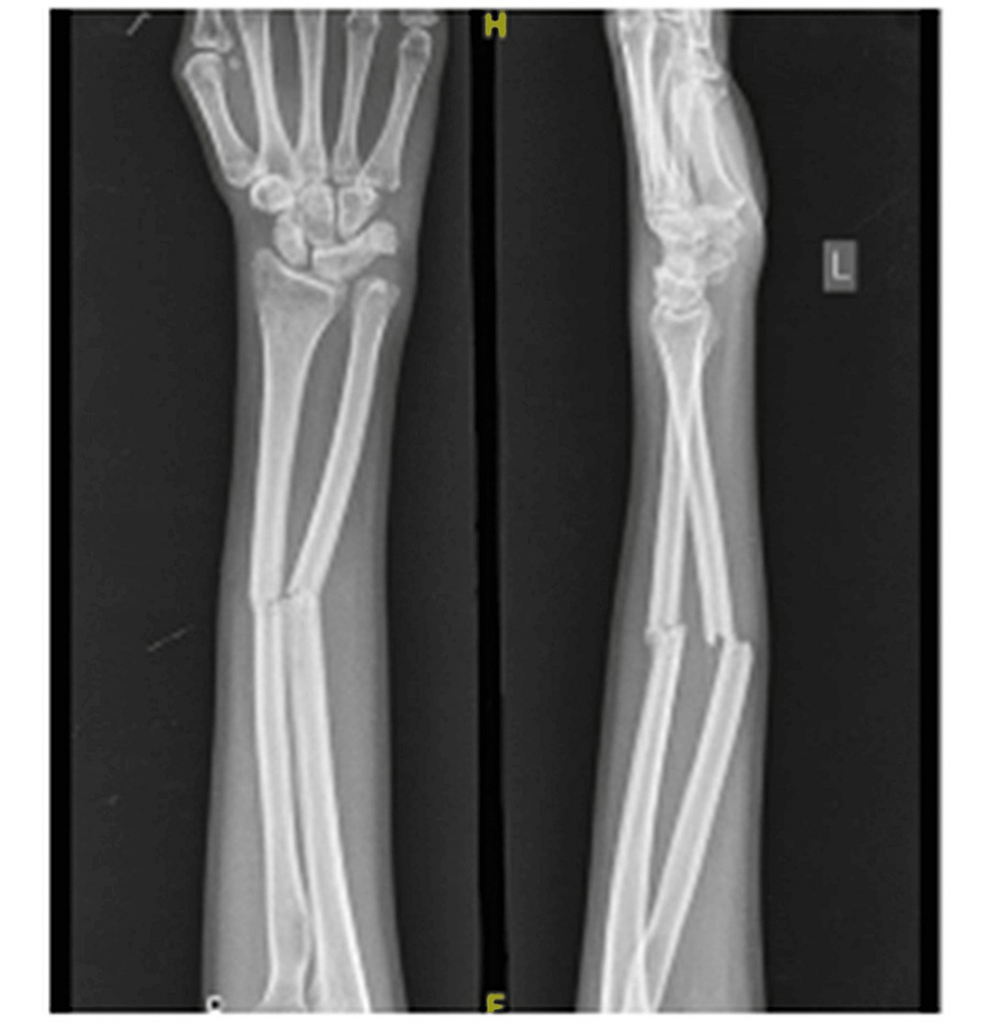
The preoperative forearm X-ray with ununited fracture of both forearm bones.
